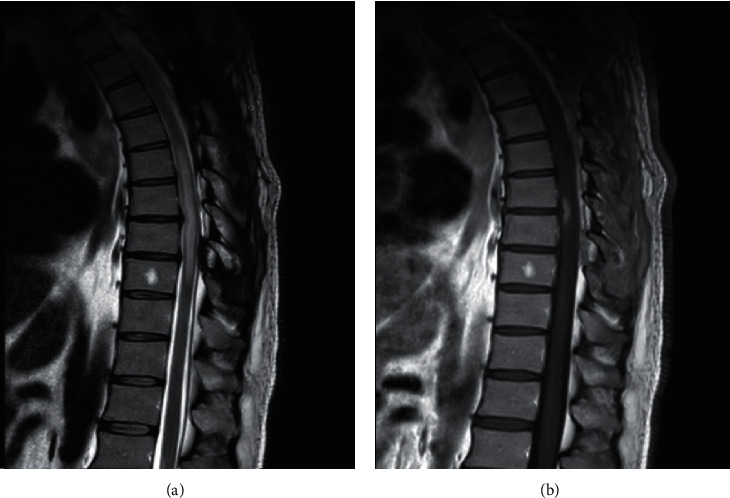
(a) Sagittal T2 MRI showing resolution of the epidural lipomatosis with resolution of the mass effect on the spinal cord and decrease in the extent of the lesion (from T6 to T9). (b) Sagittal T1 MRI with gadolinium showing mild decrease in the size of enhancing lesion.
